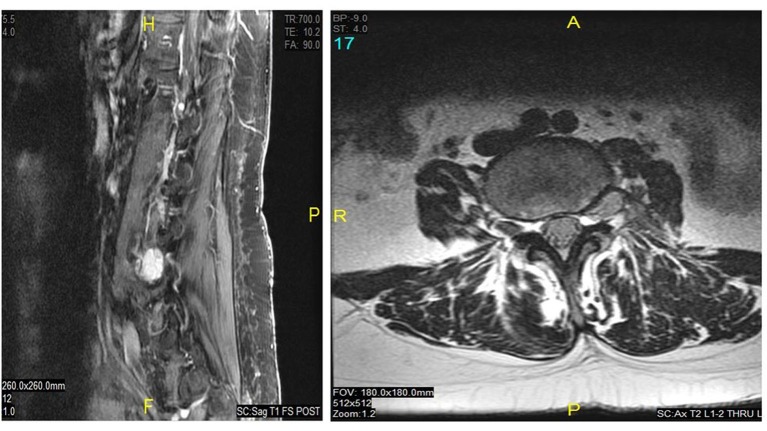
Preoperative T1 sagittal (right) and axial (left) MRI with contrast showed a variable enhancing lesion with possible fluid levels in a multilobulated lesion of the left L4 neural foramen.
